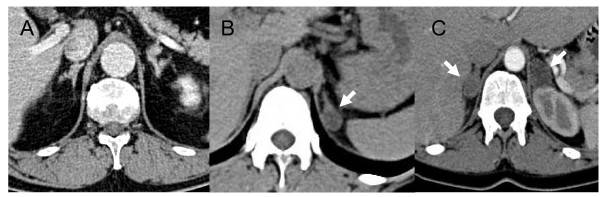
Computed tomography of the adrenals in three patients with primary aldosteronism. Note the moderate hypertrophy of both adrenal glands in A, the presence of a left hypodense nodule (arrow) in B with a thin right adrenal gland, and the presence of bilateral nodules (arrows) in C. All three aspects may coexist with uni- or bilateral aldosterone hypersecretion, which must be confirmed using adrenal vein sampling. In the patient with the computed tomography shown in C, the aldosterone to cortisol ratio was 1.6 in the inferior vena cava, 0.24 in the left adrenal vein and 42 in the right adrenal vein, indicating the presence of a right aldosterone hypersecretion associated with non-secreting left adenoma.
